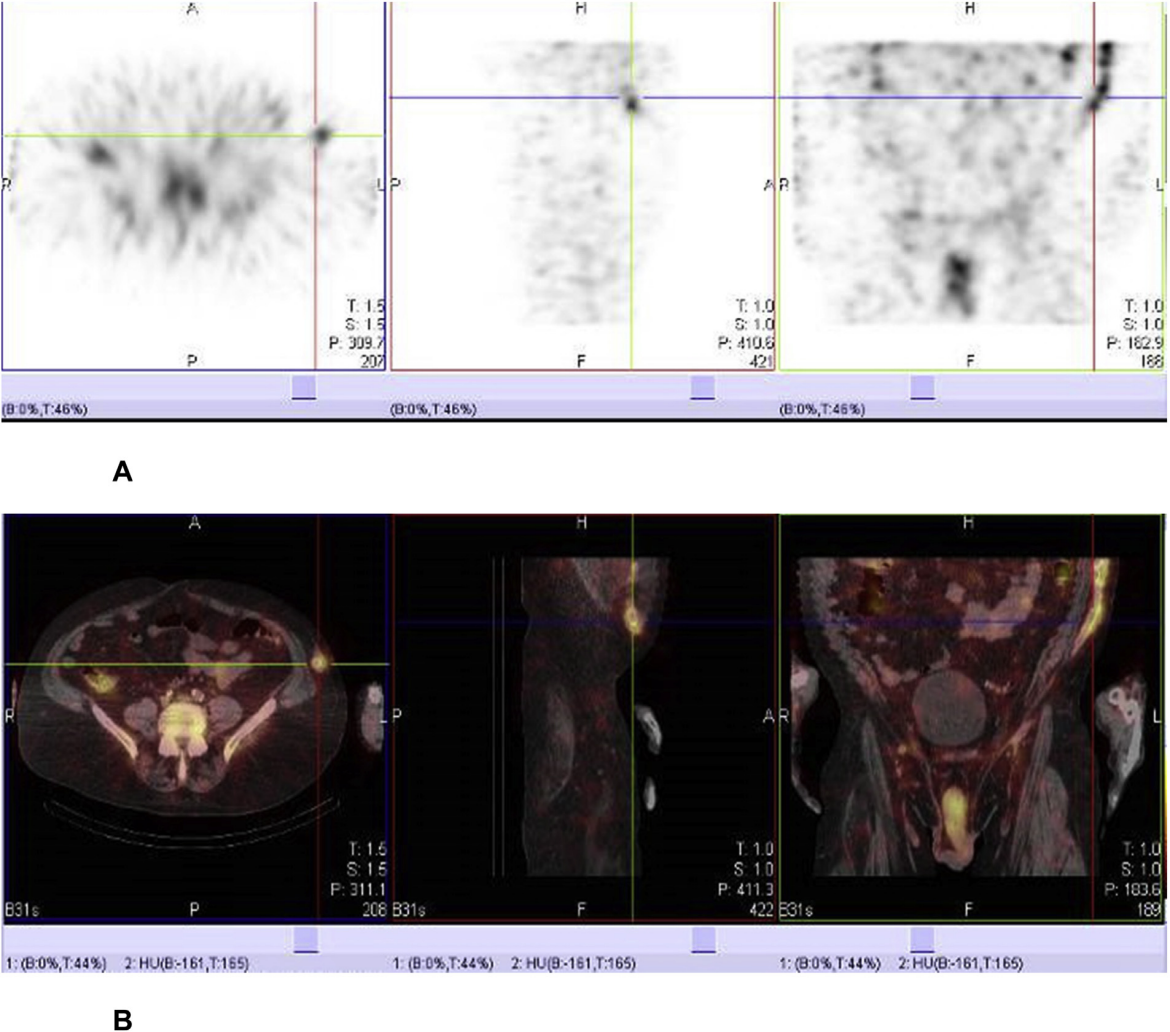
A. Multiple cross sections (*right: transverse, middle: sagittal, left: coronal views*) of an Indium-111-labeled white blood cell tagged scan showing focal increased uptake in the axillary-bifemoral graft between the spleen and pelvis suggestive of focal infection. B. Multiple cross sections (*right: transverse, middle: sagittal, left: coronal views*) of a single emission positron computed tomography-computed tomography (SPECT-CT) matching an Indium-111 labeled scan (Fig. 1A), showing focal increased uptake in the axillary-bifemoral graft between the spleen and pelvis suggestive of focal infection.
